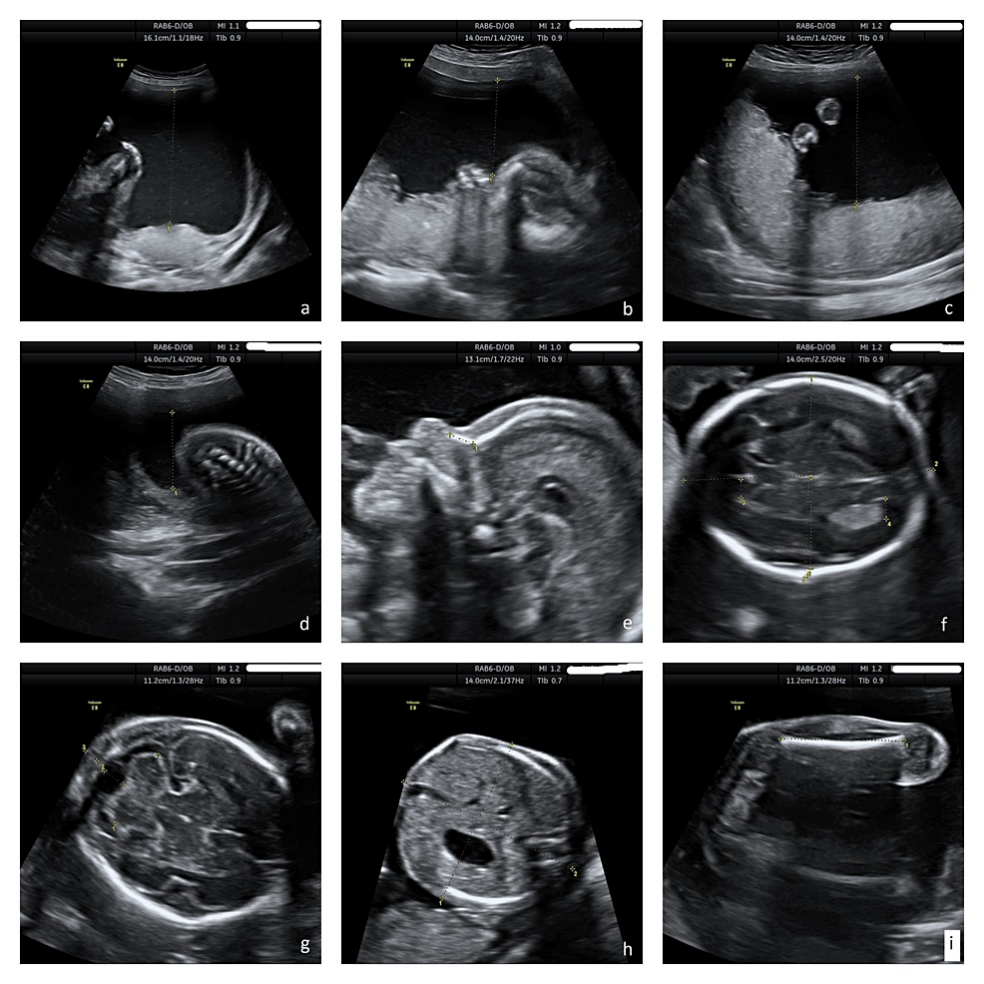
Ultrasound scan at 21 weeks demonstrates increased amniotic fluid index and a large for gestational age fetus, without any major abnormality a) First quadrant, b) second quadrant, c) third quadrant, d) fourth quadrant, e) nasal bone, f) biparietal diameter, g) occipital-frontal diameter, h) abdominal dimensions, i) femur length
